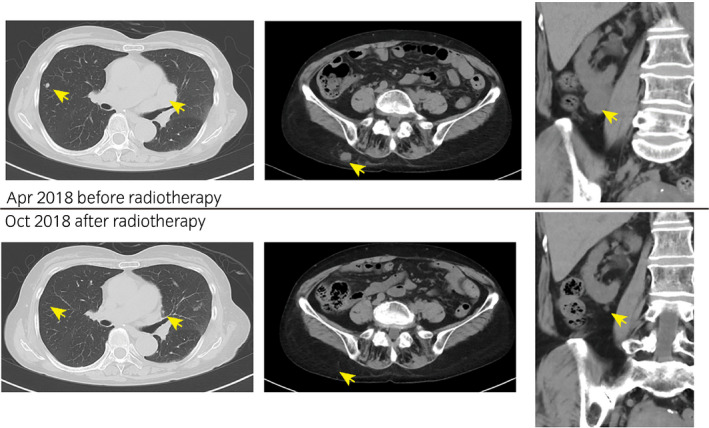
CT scan images before and after radiotherapy. The upper row shows CT scan images of lung metastases (left), subcutaneous metastasis (middle) and right renal metastasis (right) before radiotherapy (April 2018). The lower row shows CT scan images after radiotherapy (October 2018).
